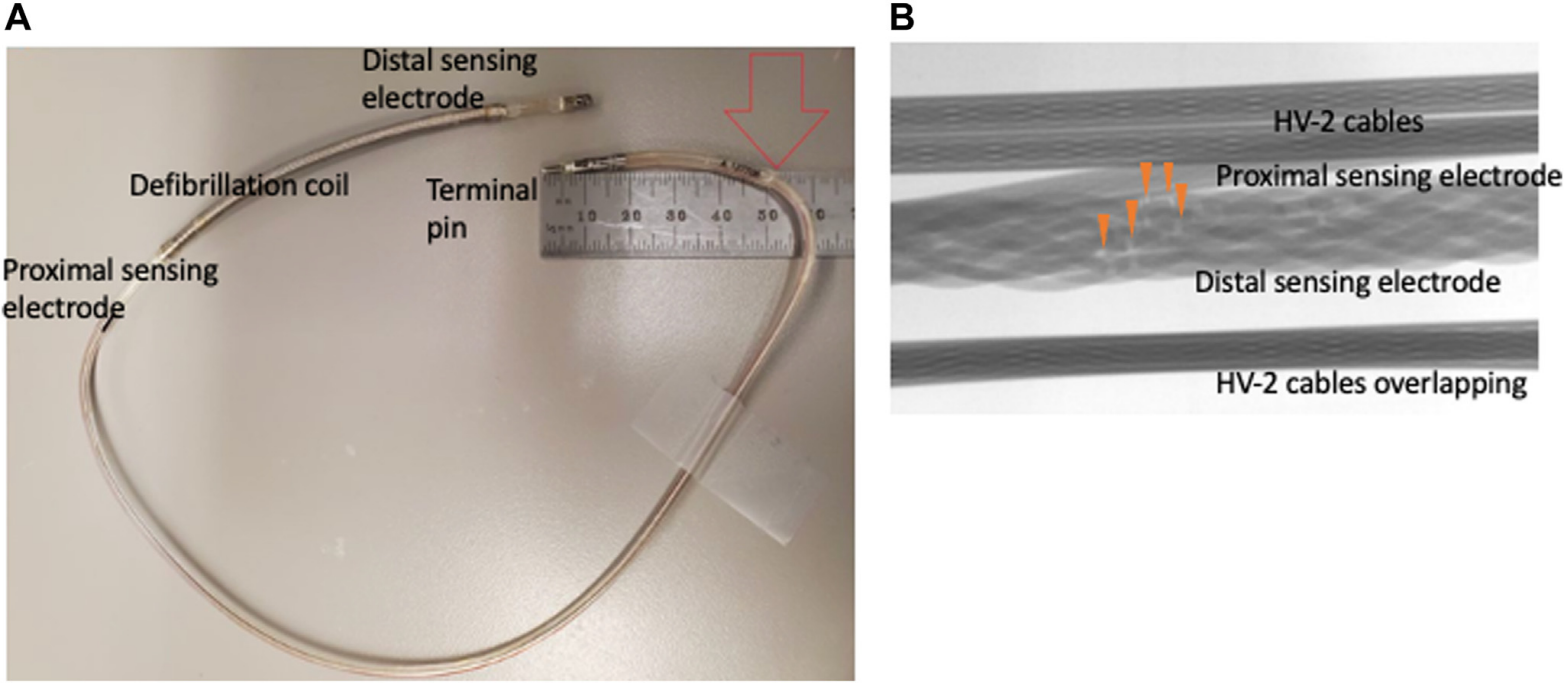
Extracted model 3401 lead showing a fracture in several filters of the distal sensing electrode. A: A bend is identified in the terminal pin area without indentation or surface abrasion. B: High-magnification radiography shows fractures in several filters of the distal sensing electrode, approximately 51 mm from the terminal pin. Yellow arrowheads indicate fractures in the electrodes.
